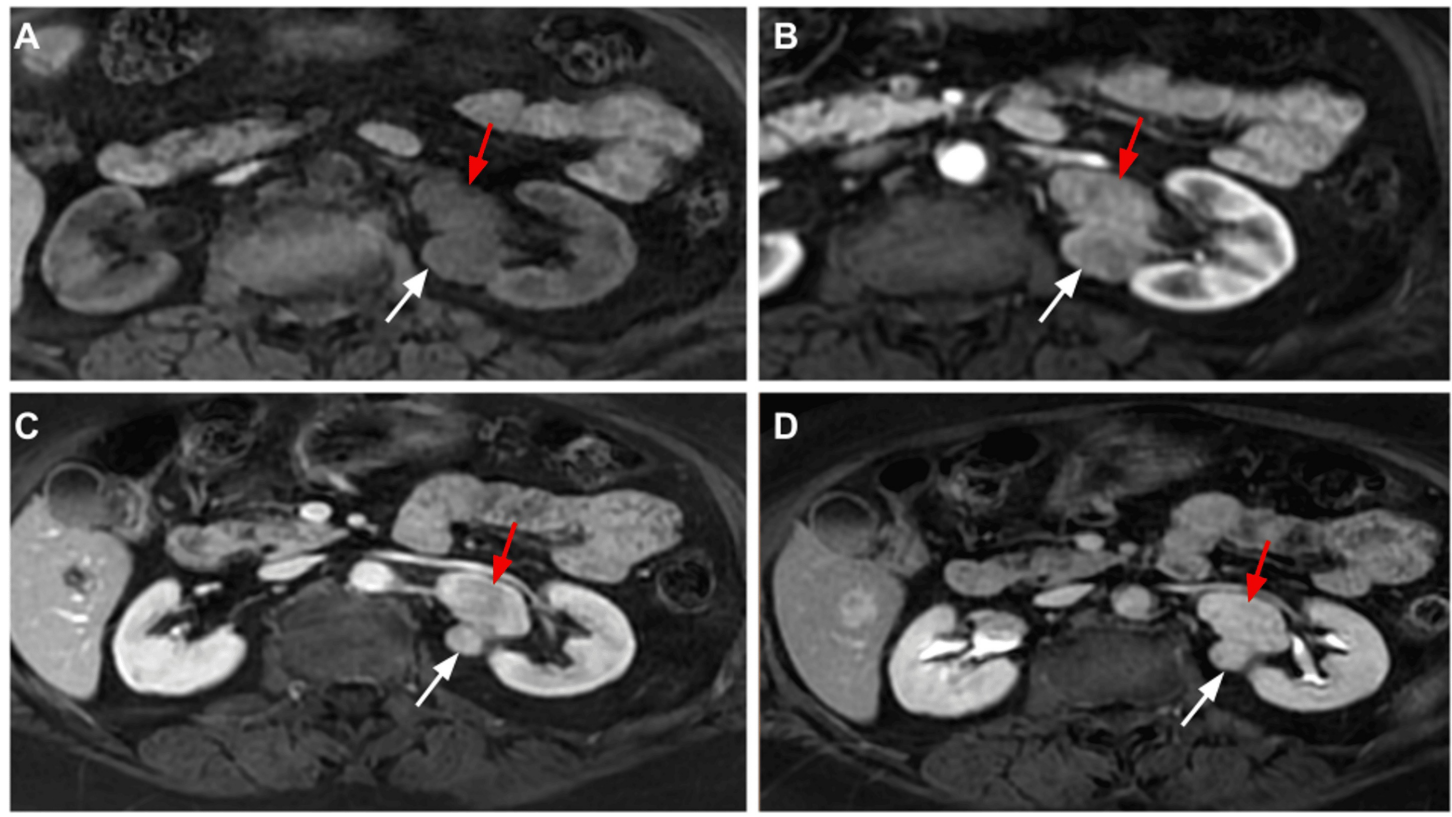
Follow up MRI abdomen three years later demonstrates relatively stable lesion that was identified previously (white arrow). And there is interval development of a new lesion within the left renal pelvis (red arrow) which has T1 intermediate signal intensity (A) and progressive enhancement of corticomedullary (B), nephrographic (C), and (D) excretory phases.
